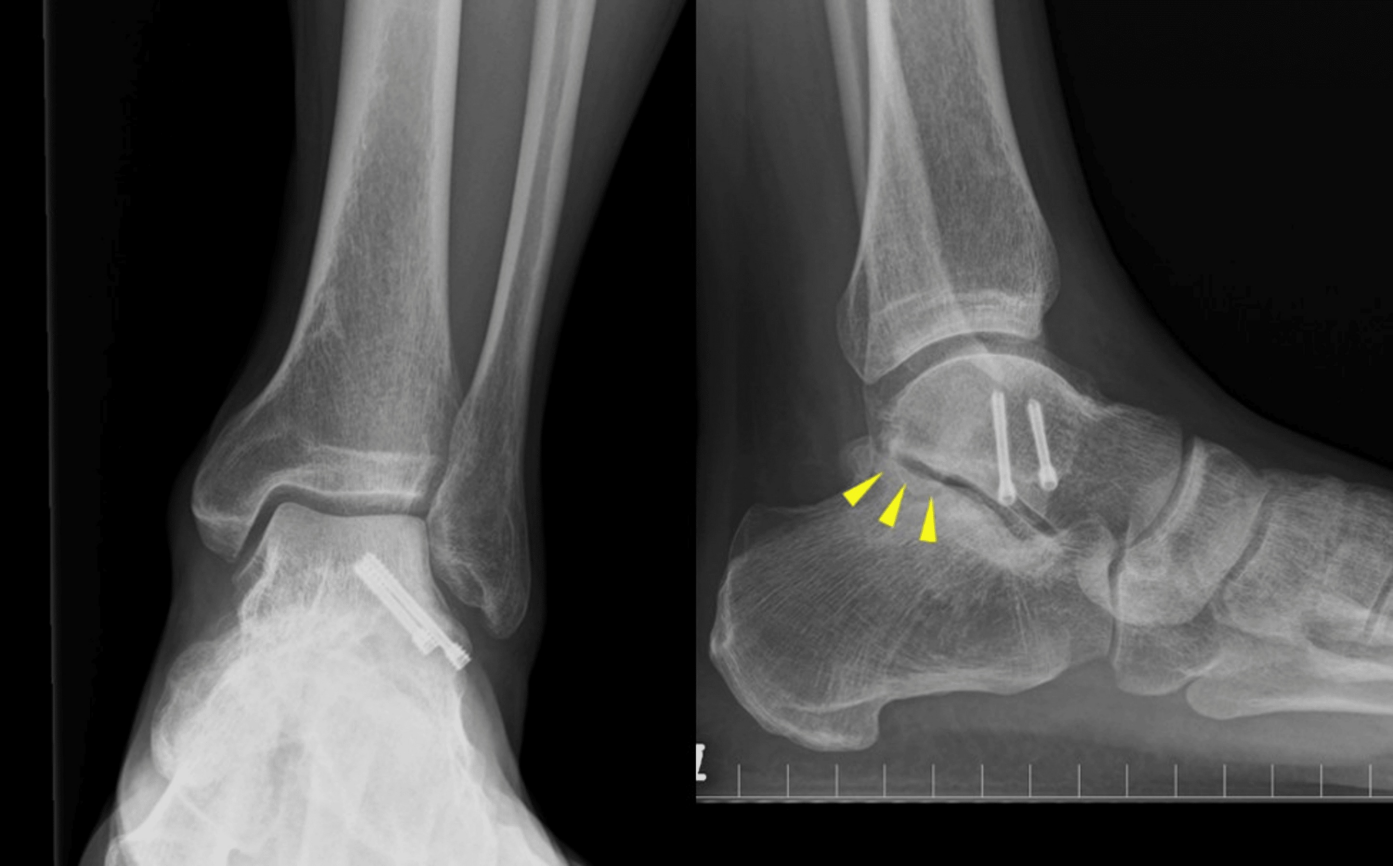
Plain radiograph findings. A: Plain radiographs at three years after surgery. Enlargement of subtalar joint space was observed.
